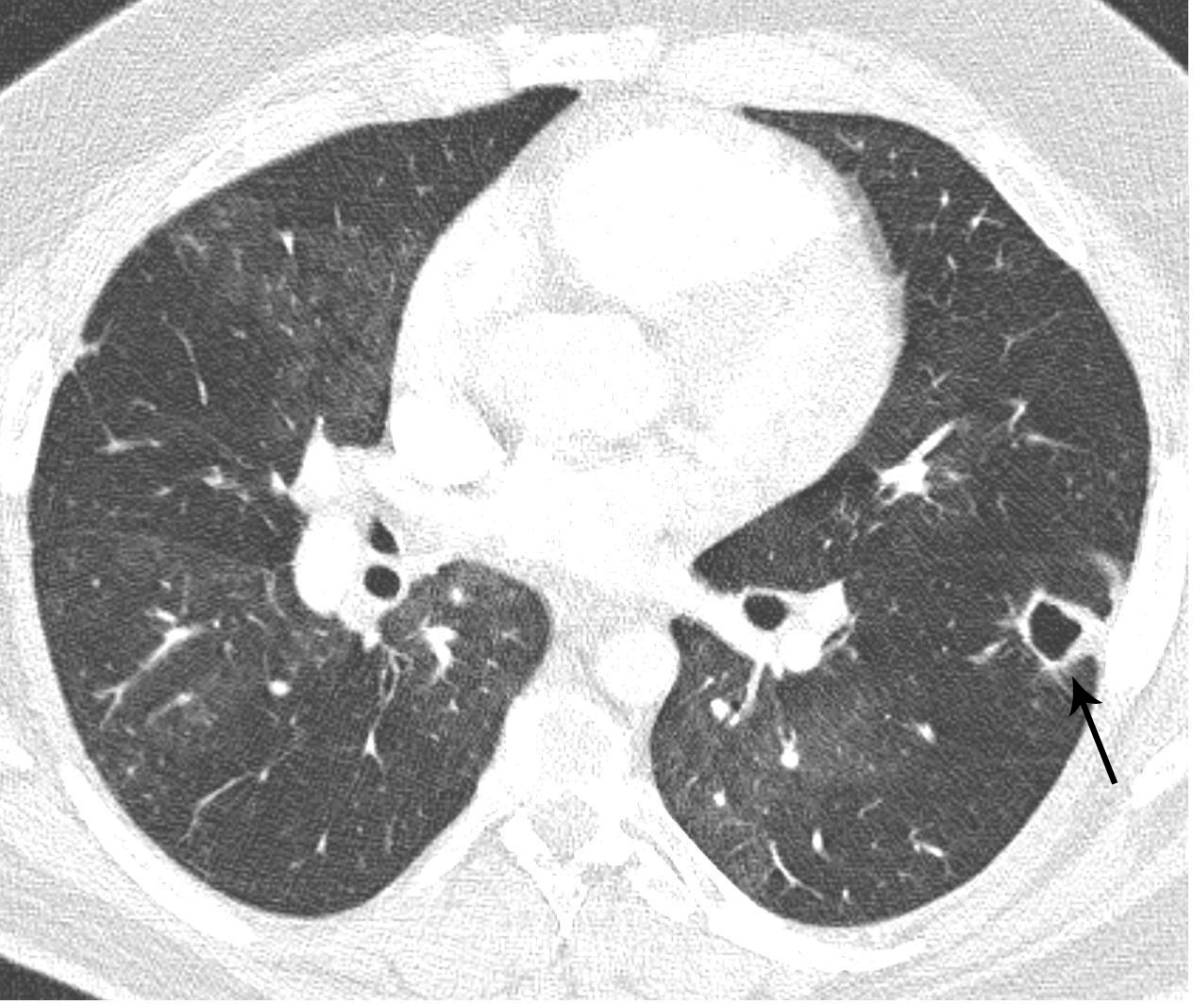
Parenchymal densities in patients with CTEPH. Axial CT image shows peripheral cavitary mass (arrow) combined with mosaic perfusion pattern 1.
